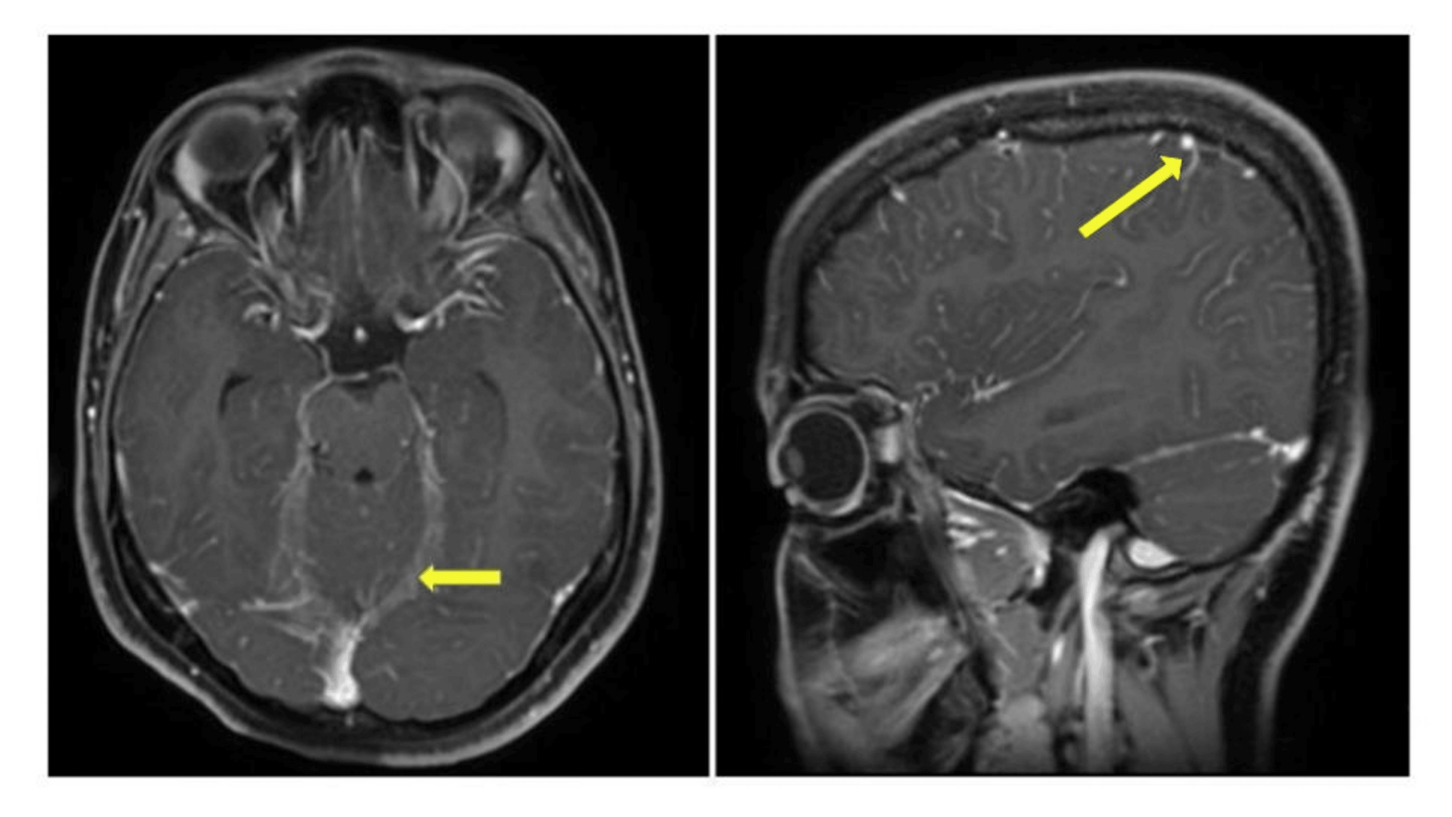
An MRI brain (axial and coronal view) showing subtle T2 FLAIR hyperintense signal intensities along the sulcal spaces of bilateral cerebral hemispheres with post-contrast mild diffuse pachymeningeal enhancement along the falx and tentorium; and leptomeningeal enhancement along sulcal spaces and cerebellar folia FLAIR: Fluid-Attenuated Inversion Recovery
